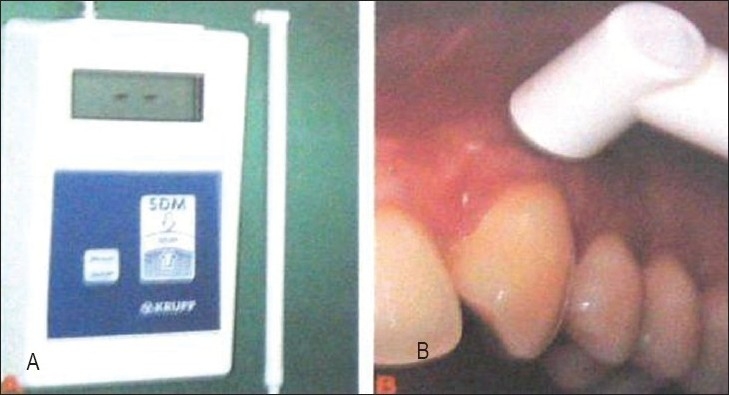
Ultrasonic device (B) measuring gingival thickness at a grafted recession site. (Muller H P, Stahl M, Eger T; 1999)
